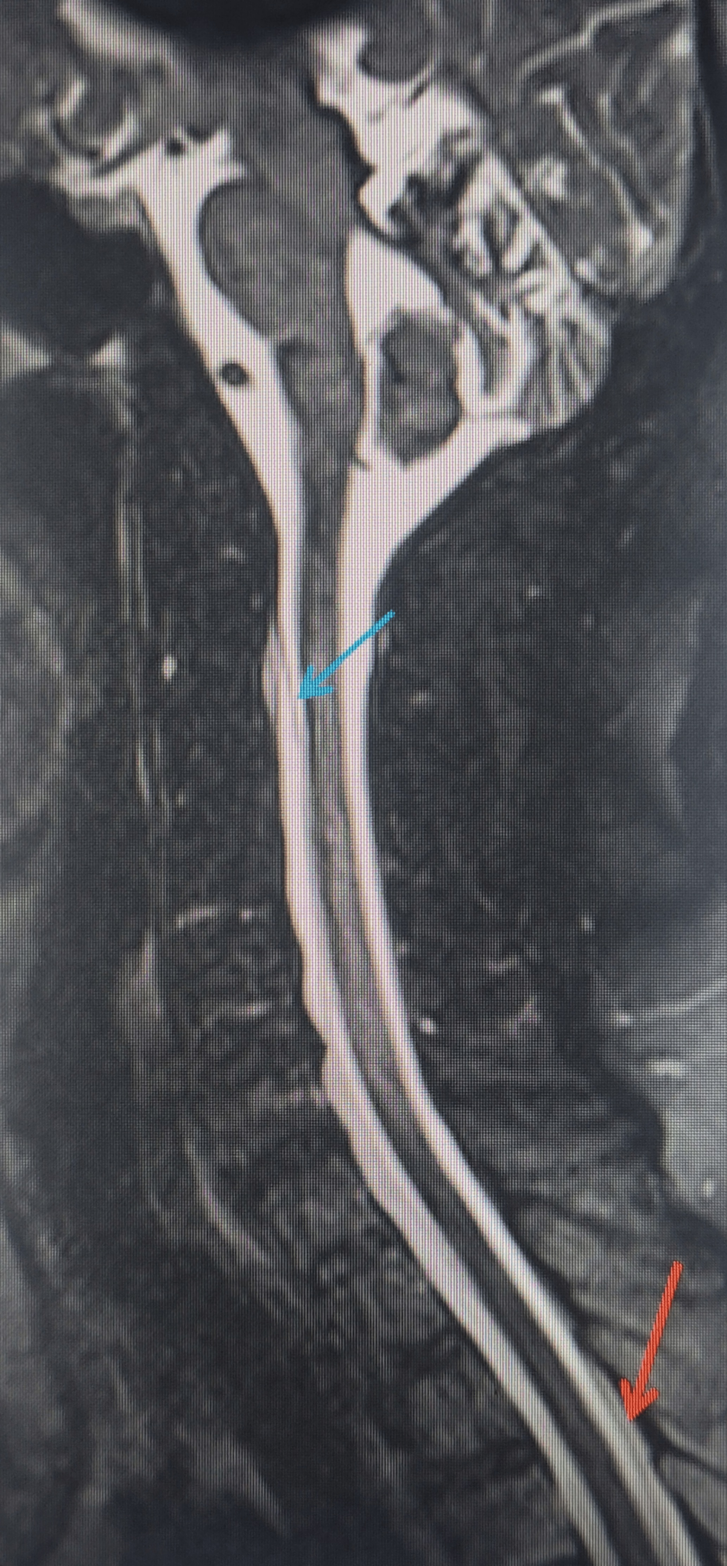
Sagittal T2-weighted fat-suppressed SPACE image of the spine showing ventral and dorsal epidural fluid collections. The image demonstrates hyperintense epidural fluid collections both ventrally (blue arrow) and dorsally (orange arrow), distinctly separated from the subarachnoid space by the intervening dural lining. SPACE: sampling perfection with application-optimized contrasts
